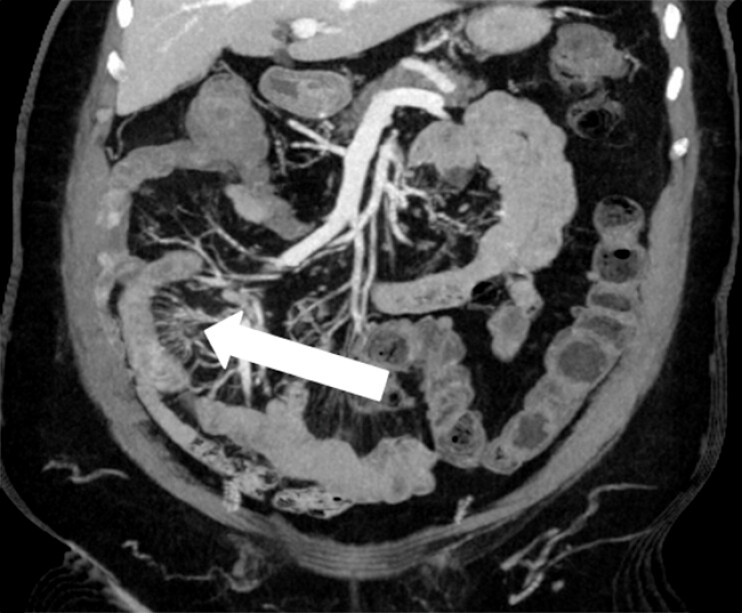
An abdominal computed tomography scan (pre-perforation) showing thickened small bowel with the “comb sign,” initially suggestive of Crohn’s disease.
